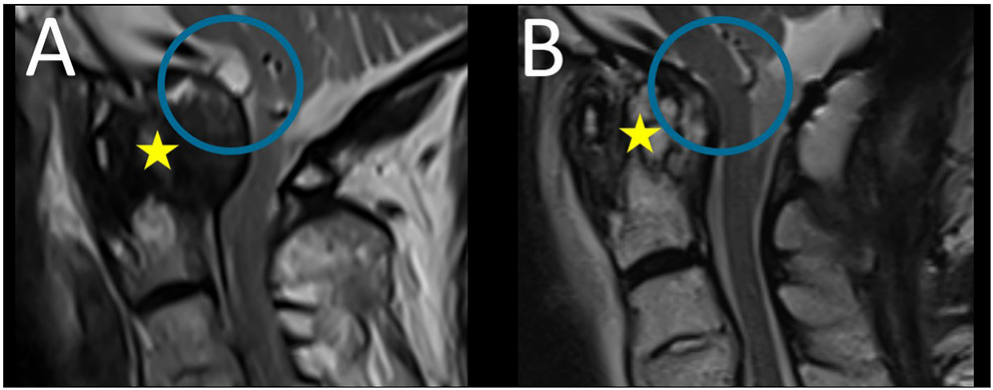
Preoperative (A) and follow-up (B) sagittal T2-weigthed magnetic resonance images: follow-up MRI scan revealed regression of the pannus (yellow star) and the cyst with replacement of the brainstem (blue circle).
